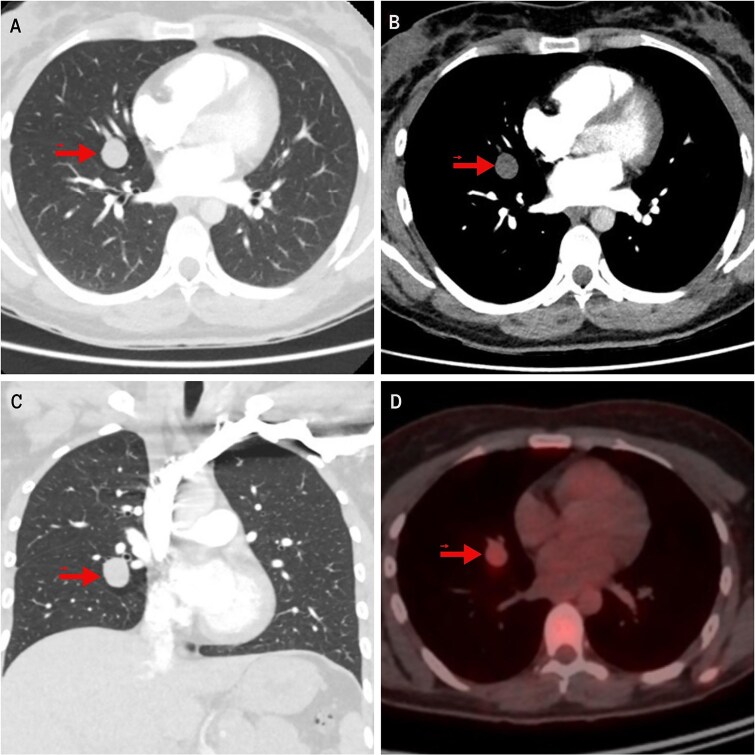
Imaging findings of the pulmonary carcinoid tumor. Contrast-enhanced chest CT. (A, B) Axial views (lung and mediastinal windows); (C) Coronal view (lung window); (D) FDG-PET scan. The arrow indicates a well-circumscribed, round lesion measuring 16 × 19 × 17 mm, with soft tissue density, centrally located in the medial segment of the right middle lobe, and showing mild hypermetabolism on image (D).
